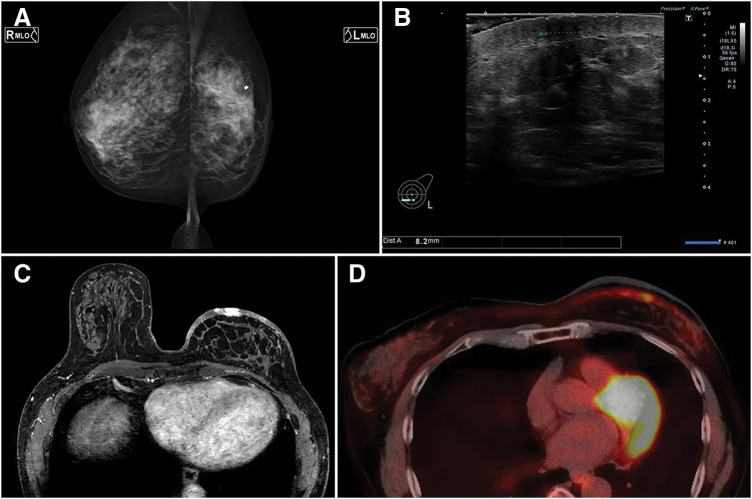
Imaging findings: (A) Mammography, (B) breast ultrasonography, (C) contrast-enhanced MRI, and (D) PET-CT.
